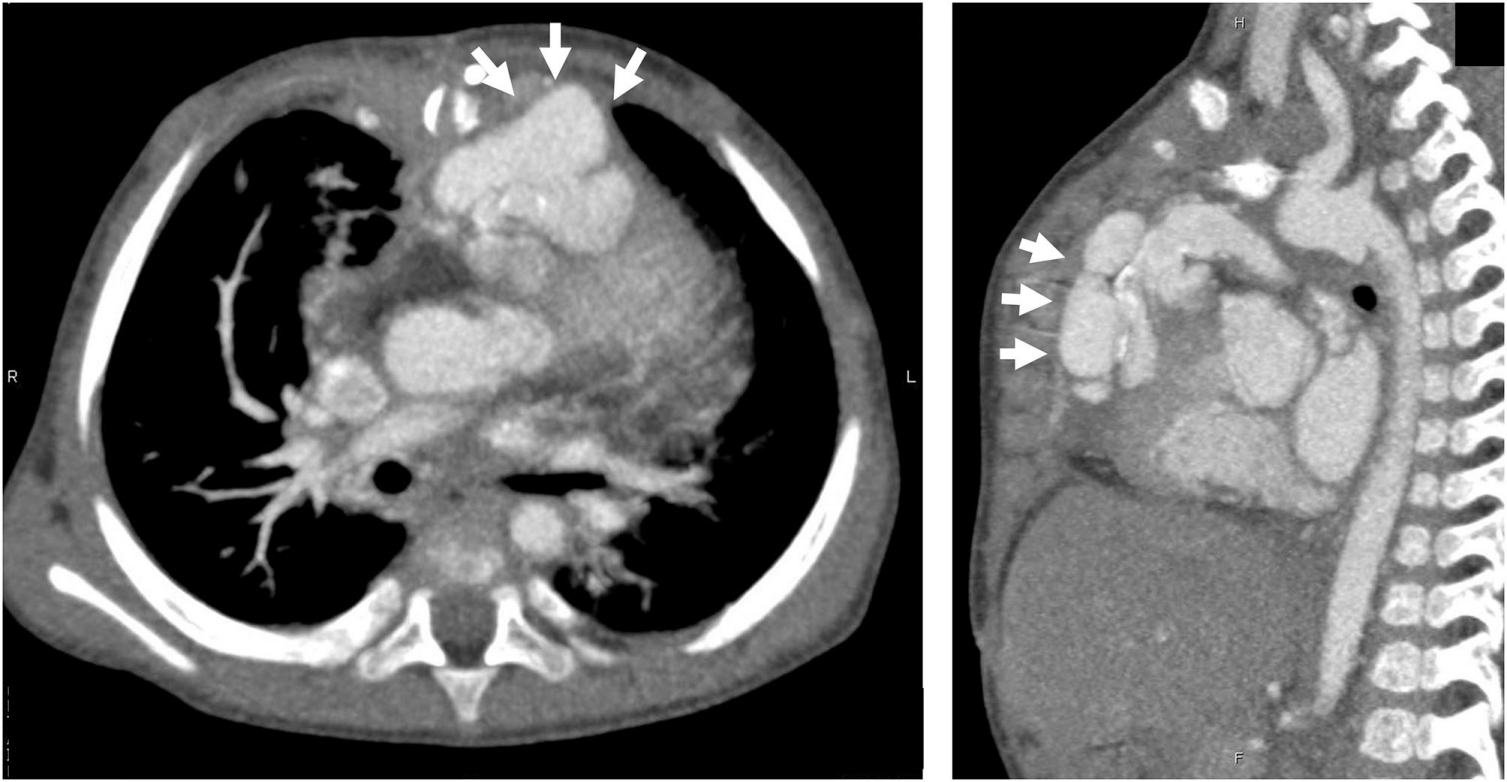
Chest CT scan at 129 days after surgery. There was a ventricular pseudoaneurysm extending from the right ventricular free wall to the posterior surface of the sternum in the mediastinum (white arrow).
